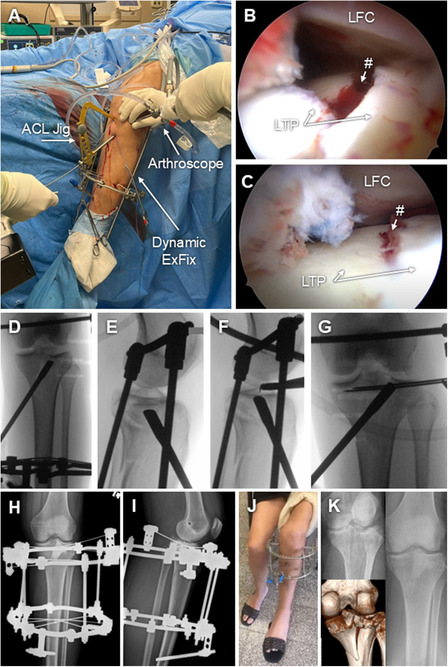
Arthroscopy‐assisted reduction of tibial plateau fracture facilitated by a dynamic external fixator. This sequence shows the same patient depicted in Figure 2A‐K, now undergoing definitive circular frame fixation with arthroscopy‐assisted reduction while still in the provisional dynamic external fixator. (A) Intraoperative image of the left knee during arthroscopy through the anterolateral portal. The dynamic fixator (Dynamic ExFix) permits knee flexion and unobstructed portal access without frame removal. An anterior cruciate ligament targeting jig (ACL Jig) is introduced to guide fracture reduction. (B) Arthroscopic view (anterolateral portal) showing a depressed lateral tibial plateau fracture line with an articular gap. (#, fracture line; LTP, lateral tibial plateau; LFC, lateral femoral condyle). (C) Same view after disimpaction and reduction of the fragment. (D) Anteroposterior fluoroscopic image of a bone tamp being inserted through the tibial tunnel created with the ACL jig. (E) Lateral fluoroscopic view showing the tamp positioned beneath the depressed lateral plateau. (F) Same view after elevation of the fragment using the tamp. Arthroscopic confirmation of the reduction is seen (scope in joint). (G) Anteroposterior fluoroscopic view showing the reduced fragment secured with 2 Kirschner wires. (H) Final anteroposterior radiograph demonstrating circular frame construct and metaphyseal void filled with tricalcium phosphate. (I) Lateral radiograph of the same final construct. (J) Photo of the final construct. (K) Top left: preoperative anteroposterior radiograph showing the index injury: a bicondylar tibial plateau fracture (Schatzker V); Bottom left: a 3D reconstruction computed tomography image of the fracture showing the depressed lateral fragment (seen from posterior view); Right: the final anteroposterior radiograph at 1‐year follow‐up.
